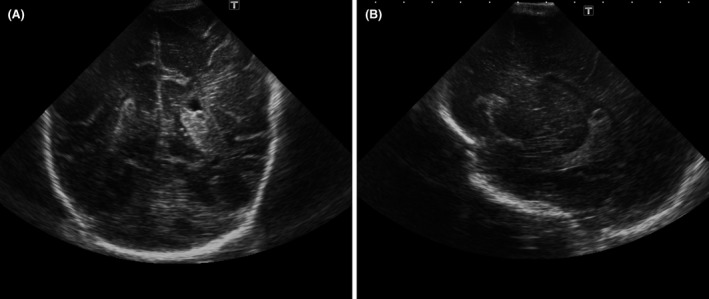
A, Coronal section of a cranial ultrasound of the patient demonstrating a bulky choroid plexus on the left side. B, Sagittal section of a cranial ultrasound of the patient demonstrating a choroid plexus cyst
